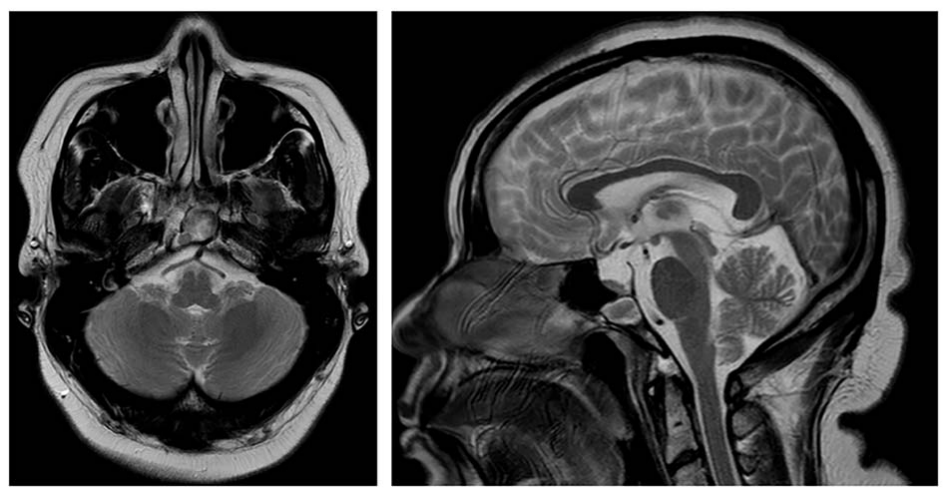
MRI IAMs (Axial T2 weighted image [T2WI] and Sagittal T2WI) showing sphenoid mucocele. The posterior clival cortex is in tact.
